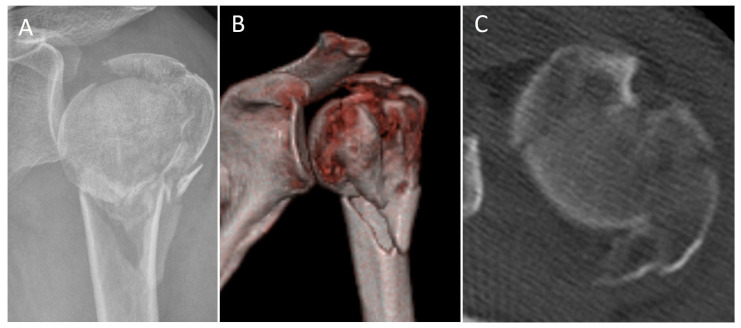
(A,B): X-ray and 3D CT-scan of a 59 year old female patient with a Resch 4GL type PHF. (C): Axial CT-scan shows the head-split of the articular surface type III accord to Scheibel et al. [9].
